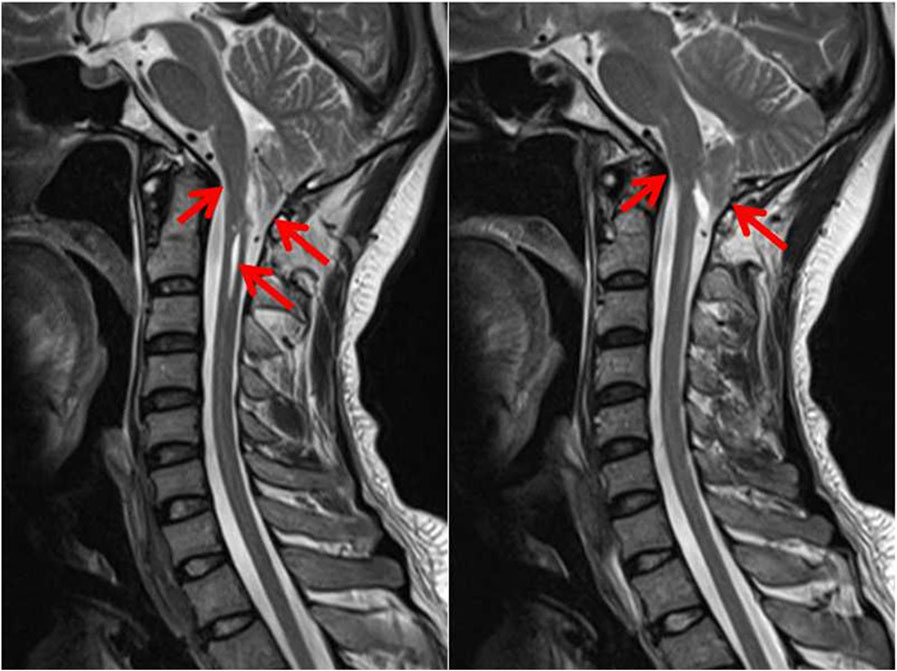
Magnetic resonance image of the cervical spine. Arrows indicate tonsillar herniation to the first cervical vertebra with a posterior dislocation of the odontoid process and syringomyelia. The medulla and high cervical cord are compressed and deformed
